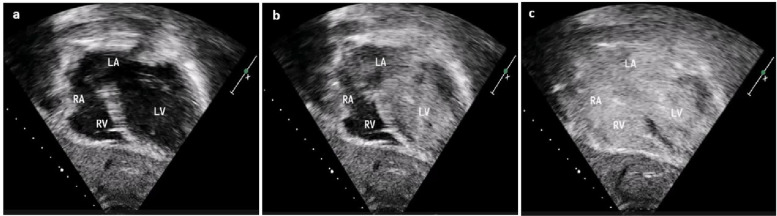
(A–C) Saline contrast echocardiography with series of images in a different patient. Subcostal four-chamber view after left arm contrast injection, demonstrating contrast bubbles filling left atrium (LA), left ventricle (LV), right atrium (RA), and right ventricle (RV), suggestive of completely unroofed coronary sinus with persistent left superior vena cava
